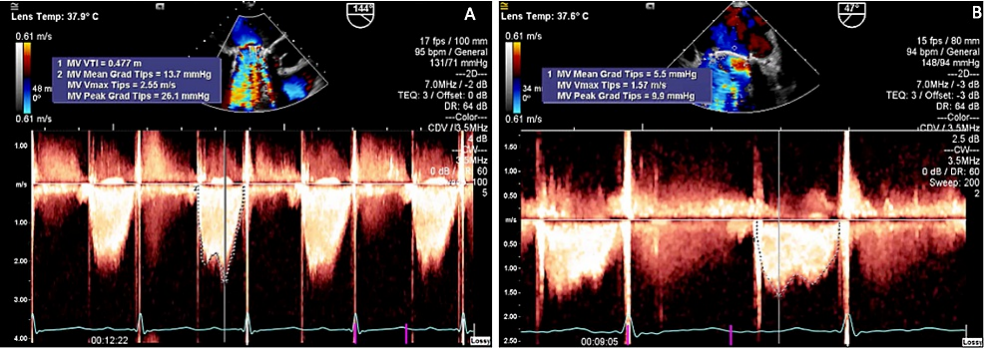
Transesophageal Doppler echocardiogram demonstrating normalization of trans-prosthetic mean gradient after thrombolysis with ultraslow high-dose t-PA Transesophageal Doppler echocardiogram showing on the left (A) a trans-prosthetic mitral mean gradient of 13.7 mmHg at a heart rate of 95 beats per minute prior to thrombolysis with the ultraslow high-dose t-PA; on the right (B) notice normalization of the trans-prosthetic mitral mean gradient (5.5 mmHg) at a heart rate of 94 beats per minute after thrombolysis with the ultraslow high-dose t-PA. t-PA=tissue plasminogen activator, MV=mitral valve
